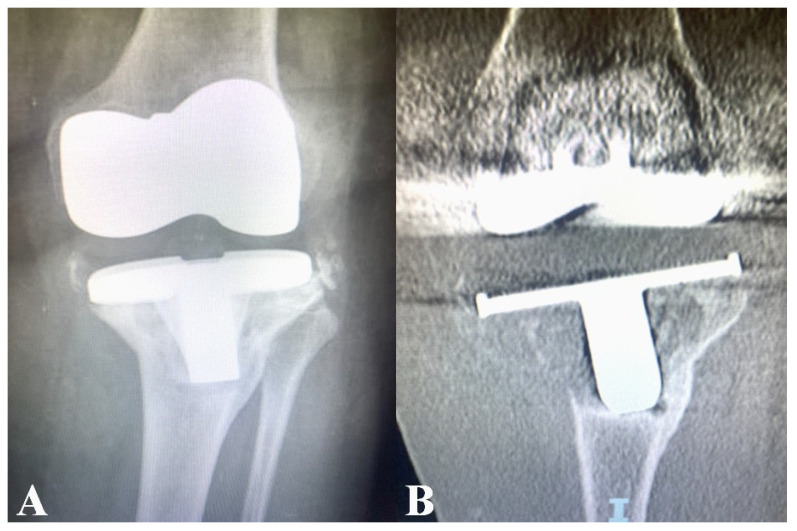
(A,B) Patient’s X-ray and CT scan of left knee showing implant loosening.
